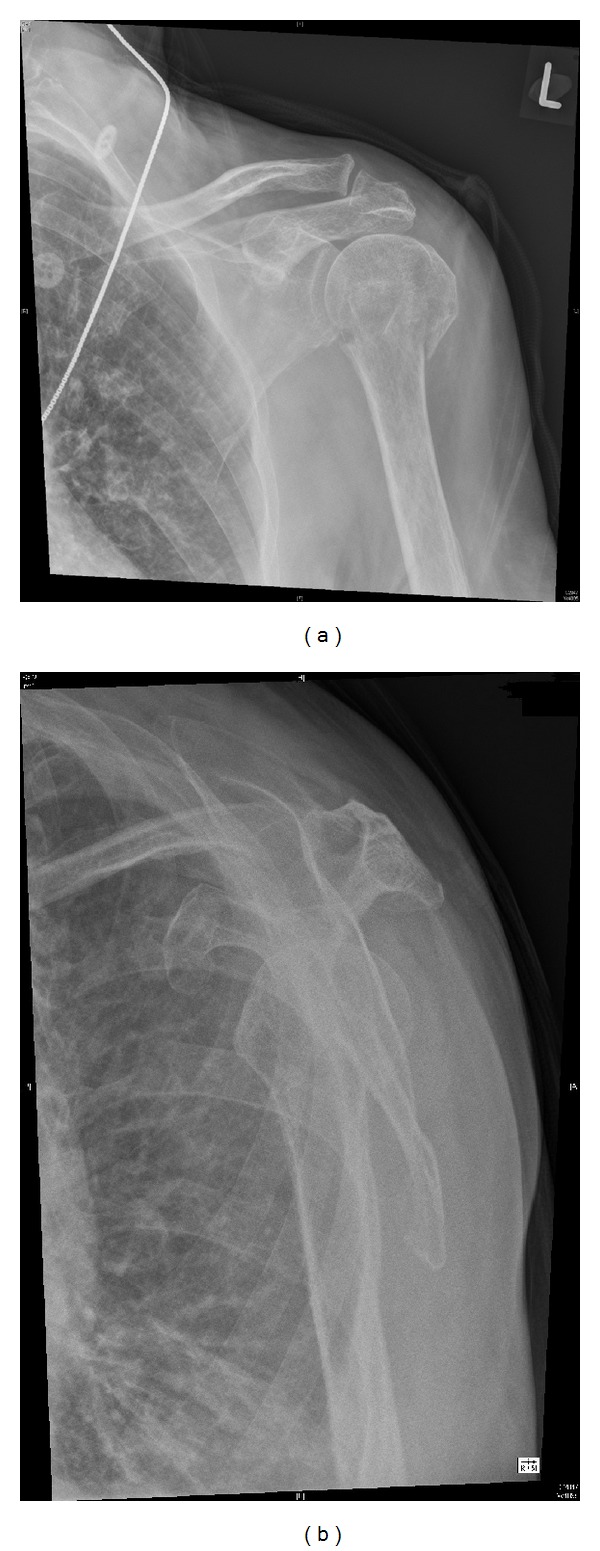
AP and lateral plain film radiographs demonstrating a proximal humerus fracture.
